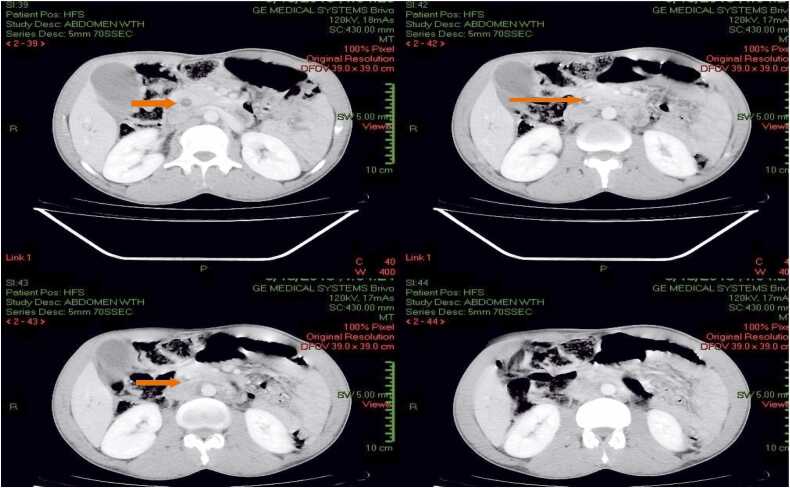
CT Scan: Distended GBD, CBD, and Continuous Hyper-dense structure with central hypo-density (Slice 39–44). The red arrow indicating the filling defect within the CBD.
